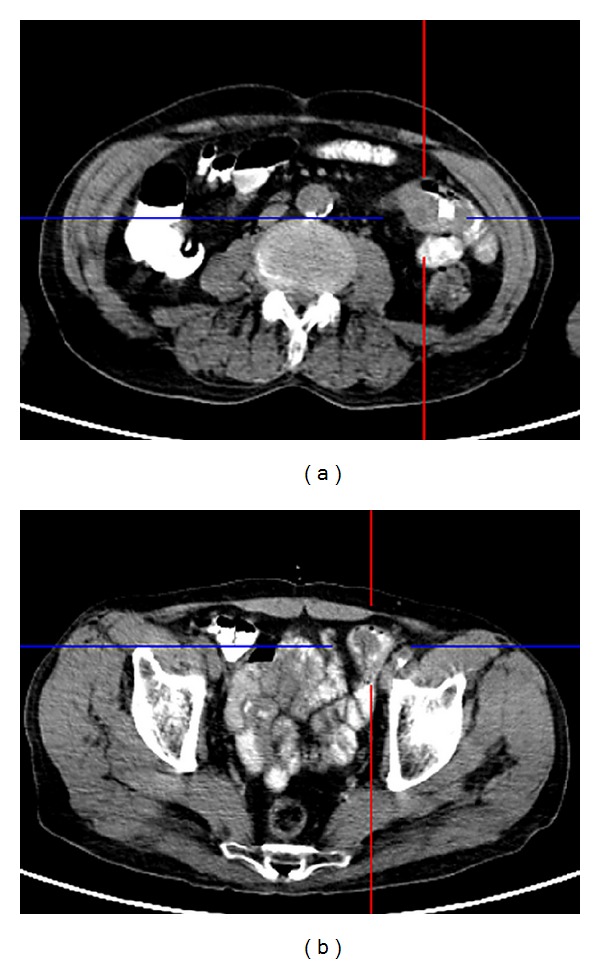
Abdominal CT image of tumors located in the small intestines.
